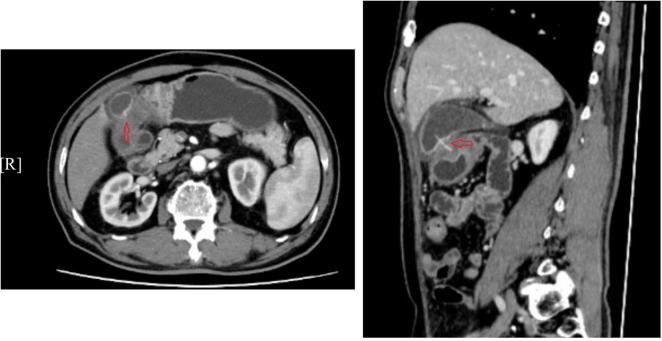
Abdominal CT scan showing a 3 cm curvilinear hyperdense foreign body (red arrow) positioned between the gallbladder cavity and gastric antrum, with surrounding inflammation.
